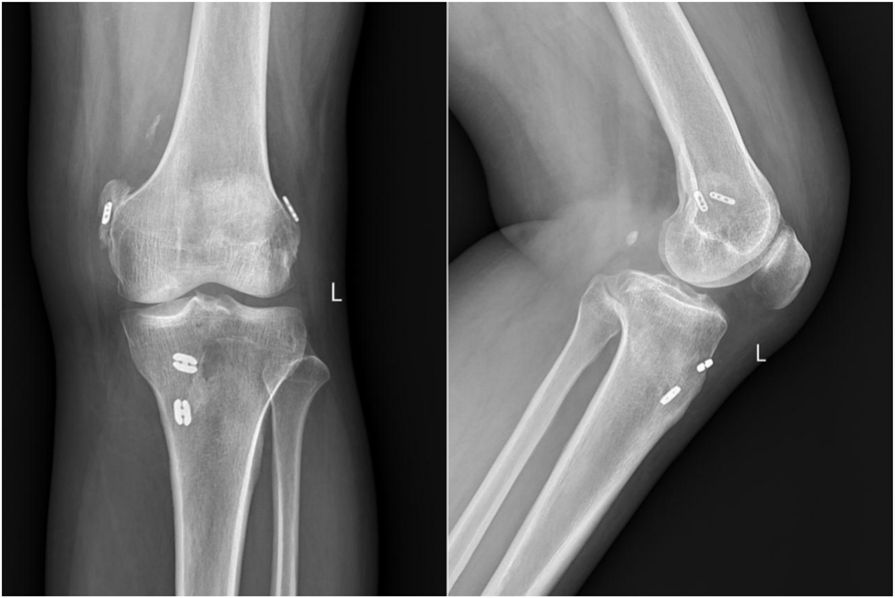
Radiographs of the knee in adult-onset still's disease. Postoperative changes of the combined ACL and PCL reconstruction with good internal fixation position
